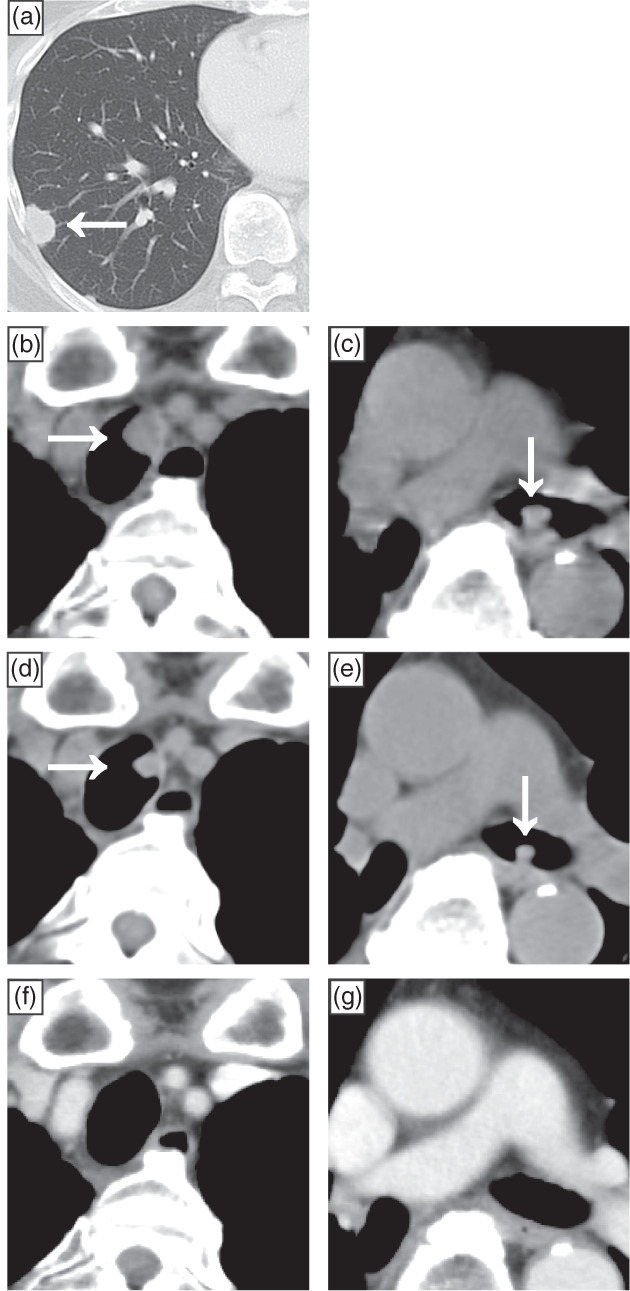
Before surgery, computed tomography (CT) showed a nodule in the lower lobe of the right lung (a). Eleven months after thoracoscopic lower lobectomy of the right lung, CT showed nodules in the trachea (b) and left main bronchus (c). CT performed 6 days after the first course of first‐line chemotherapy revealed that these nodules had shrunk (d, e). CT after four courses of first‐line chemotherapy (with the addition of accelerated hyperfractionated radiotherapy starting with the third course) showed that the nodules in the trachea and left main bronchus had disappeared (f, g).
